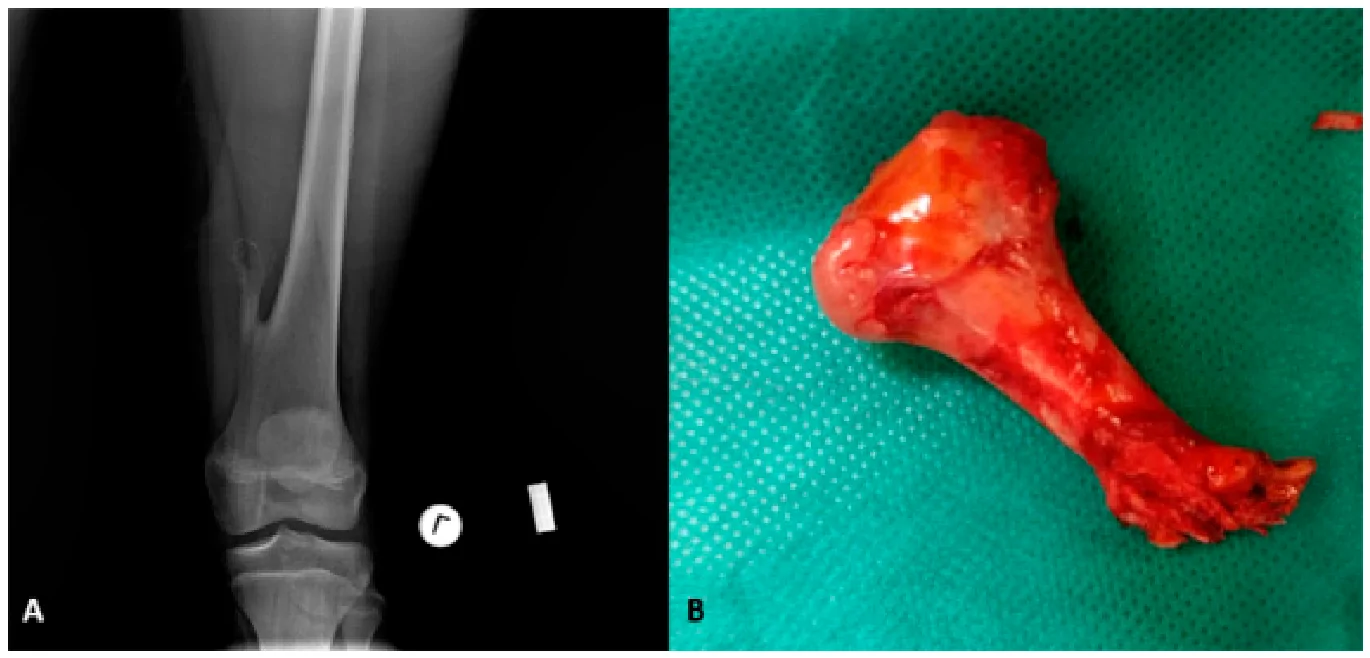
Osteochondroma of the distal femur in a 12-year-old girl. (A) Preoperative radiograph demonstrating a well-defined exophytic bone lesion arising from the distal femur; (B) Intraoperative view showing the lesion after surgical exposure and complete excision.
